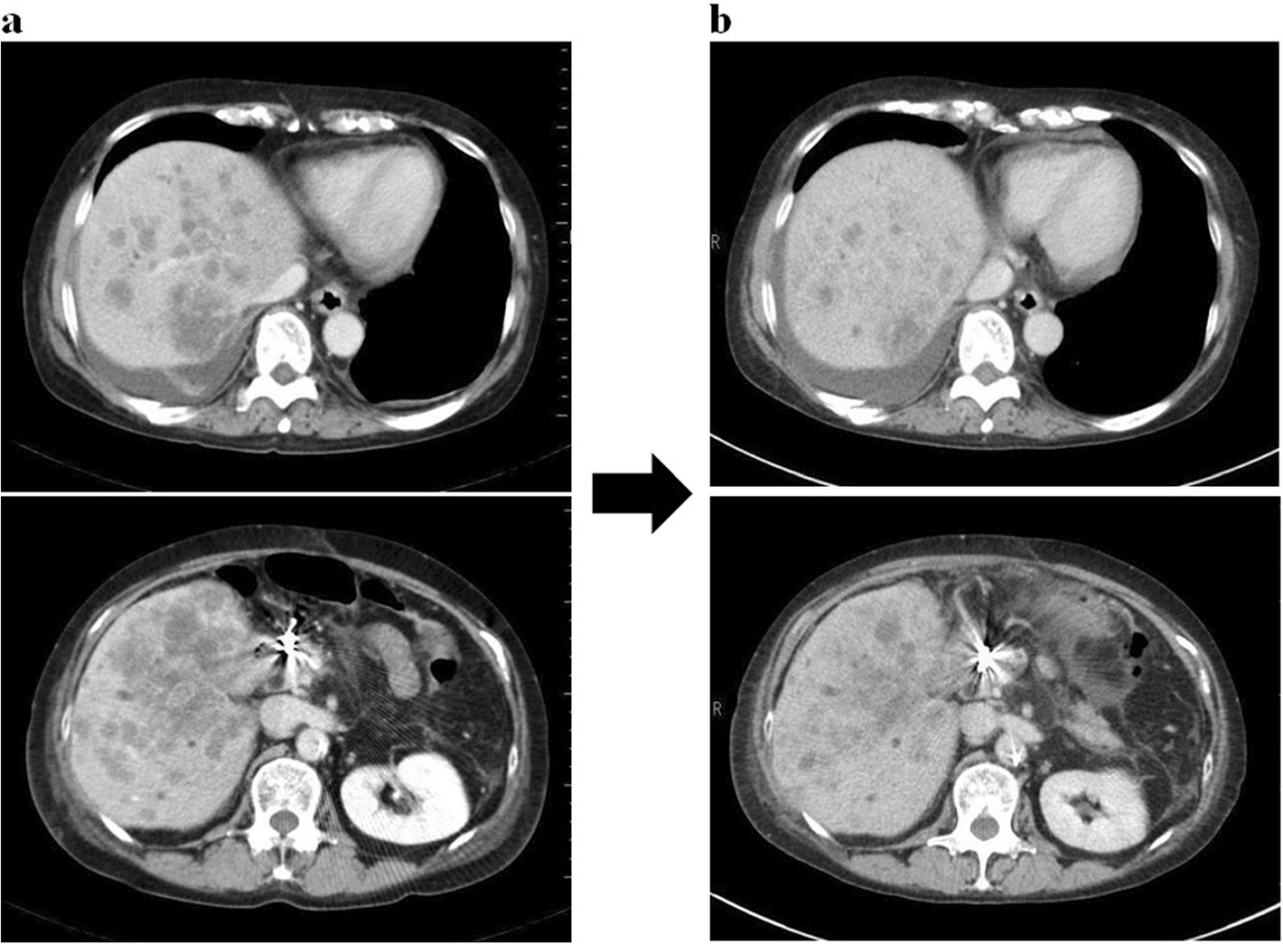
Abdominal computed tomography. (a) Before treatment. Metastatic lesions in both lobes of the liver were revealed. (b) After two courses of treatment, hepatic metastasis was decreased: The metastatic lesion in the liver had reduced, and the other lesions also tended to be reduced in size.
